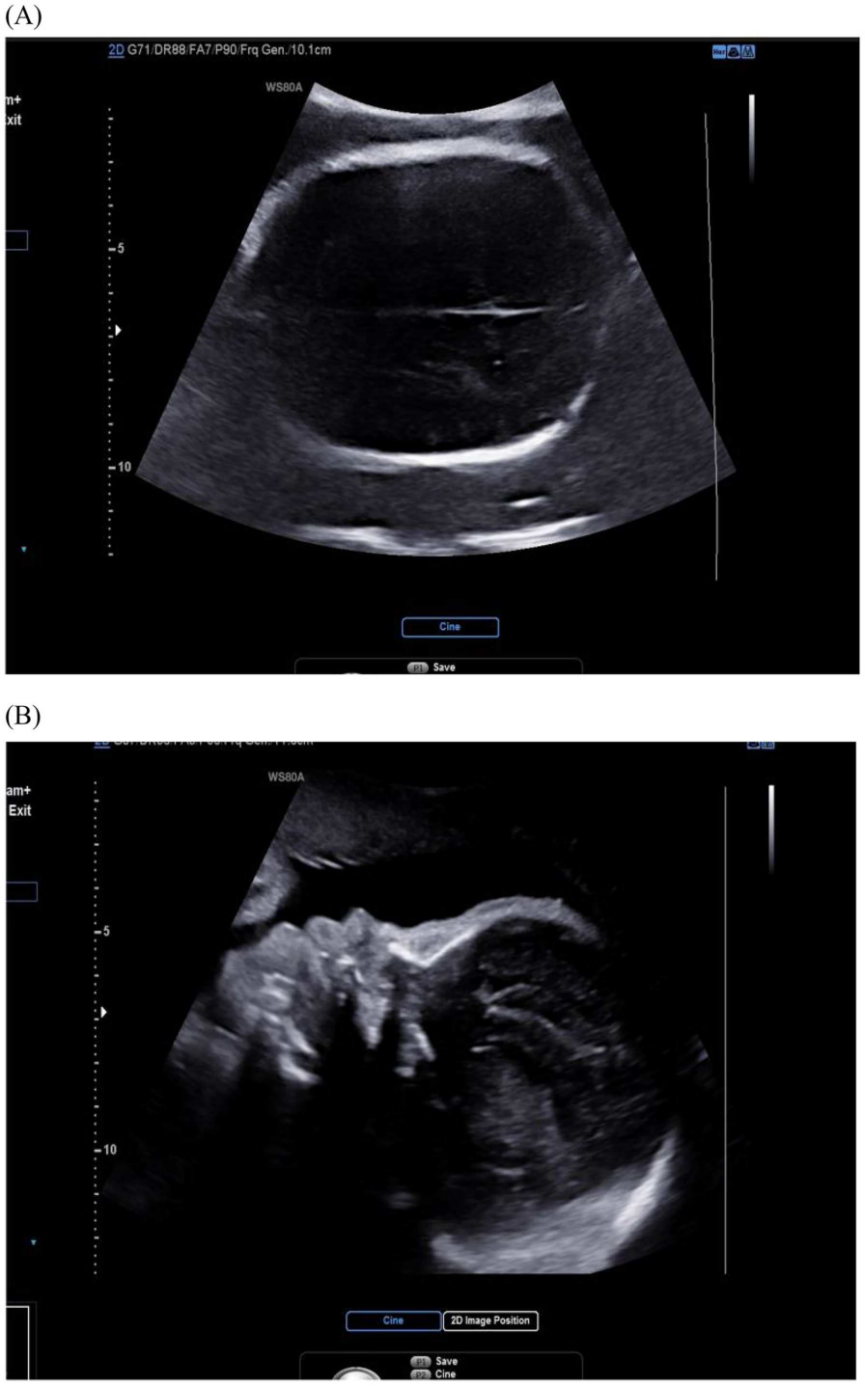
Ultrasound image of fetal skull in Apert syndrome: closed coronal suture (cross-section) and frontal bossing. (A) Cross-section view: the coronal suture, as seen in the cross-section of the skull, is closed and therefore not visible; (B) midsagittal view: the skull shape is abnormal, with a protruding forehead.
